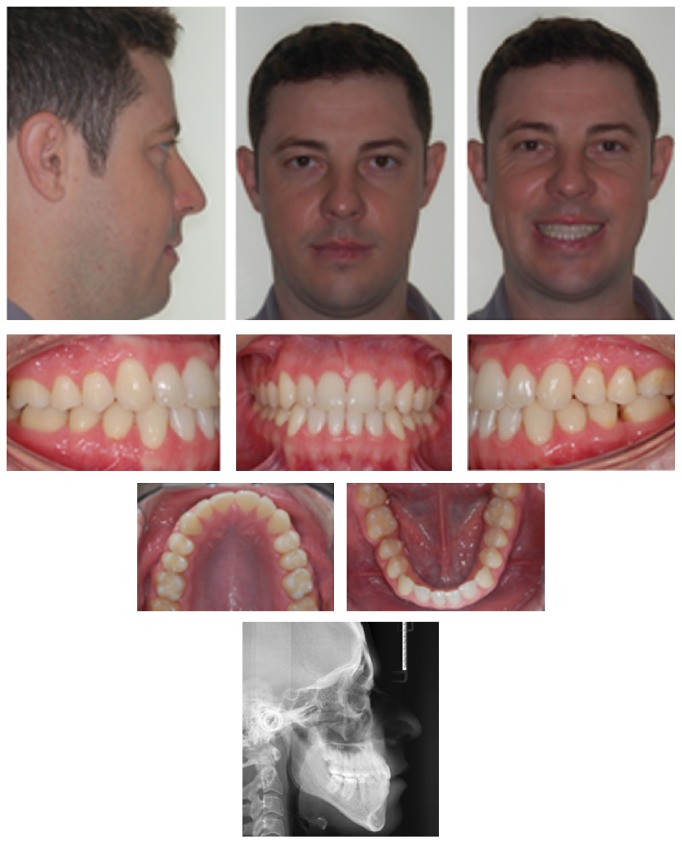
Posttreatment facial and intraoral photographs, and cephalometric radiograph (2 years after treatment completion).
